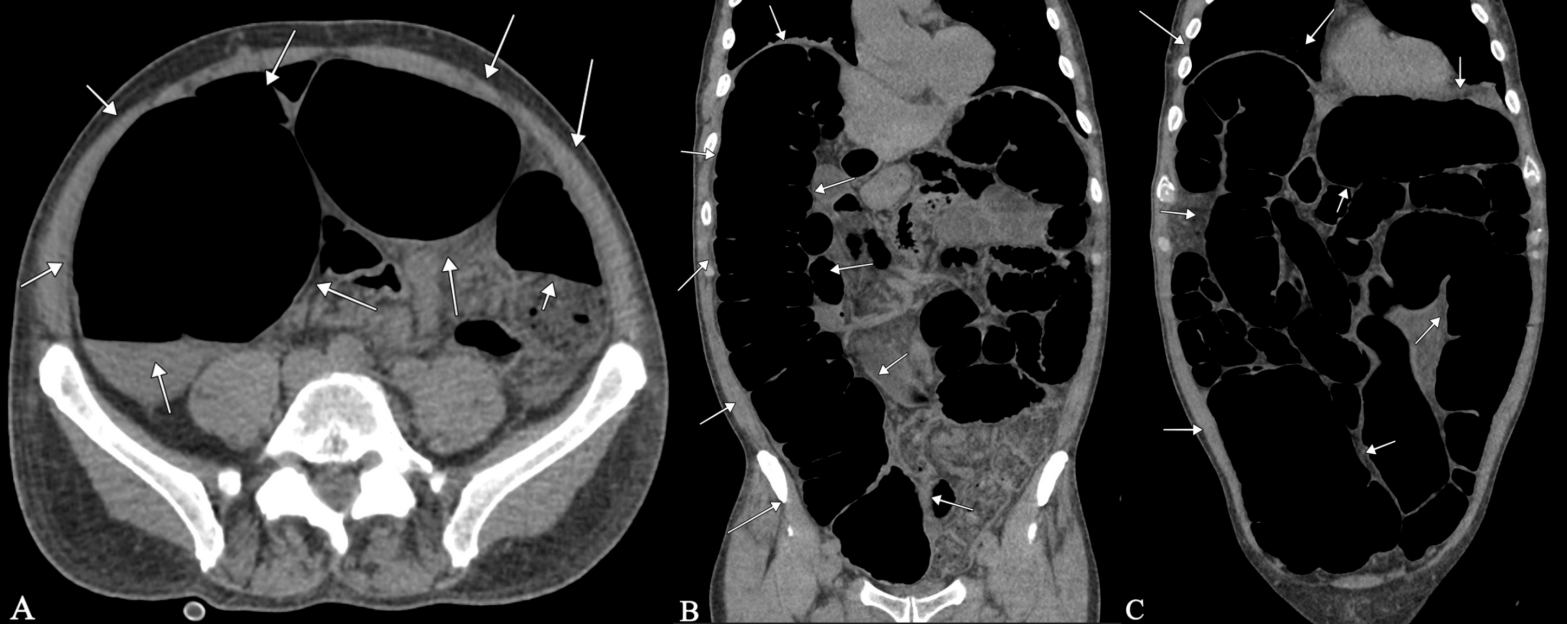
Non-contrast abdominal computed tomography (CT) scan showing severe colonic dilatation. (A) Axial view demonstrating marked dilatation of the ascending colon, with the cecal base indicated by white arrows, measuring approximately 14 cm.
